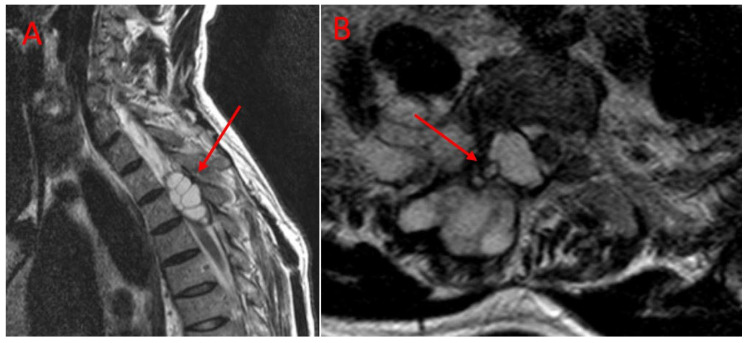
Preoperative T2-weighted MR images of a patient treated with CLDS. (A): The arrows indicate multilocular hydatid cysts invading the spinal canal. (B): The arrows indicate hydatid cysts (shown from an axial perspective) with a unilateral location extending into the spinal canal and paravertebral muscles.
